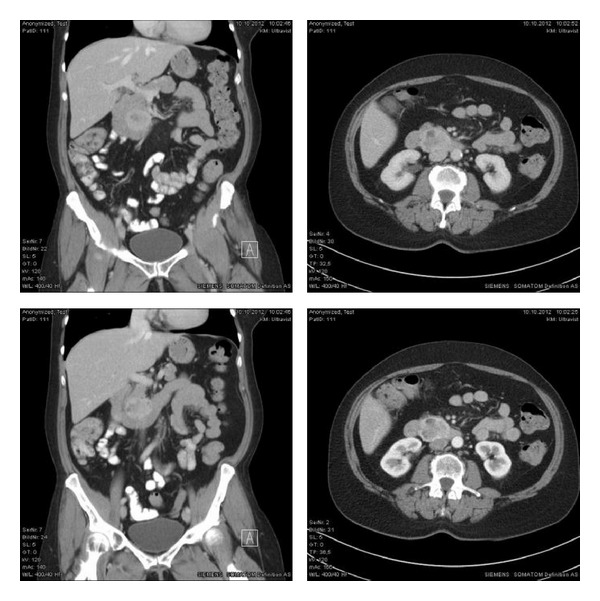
CT-scan of the abdomen with the pancreatic mass of enhancing and nonenhancing areas.
